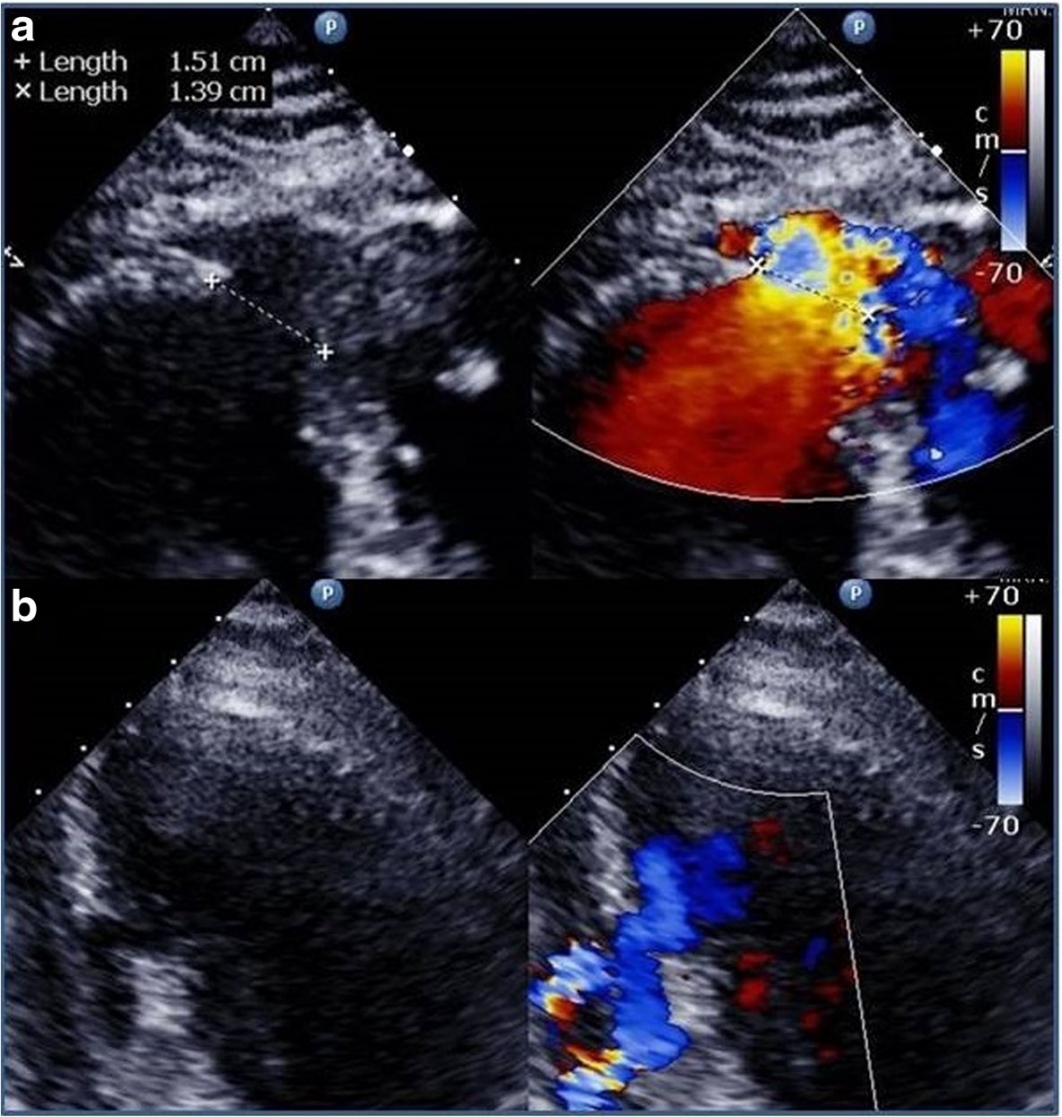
Preoperative echocardiographic findings. a TTE in four chamber view showing a 15 mm VSD in the apicoanterior ventricular septum with left to right shunt. b Color flow Doppler image showing the 8 mm VSD in the mid anterior ventricular septum
